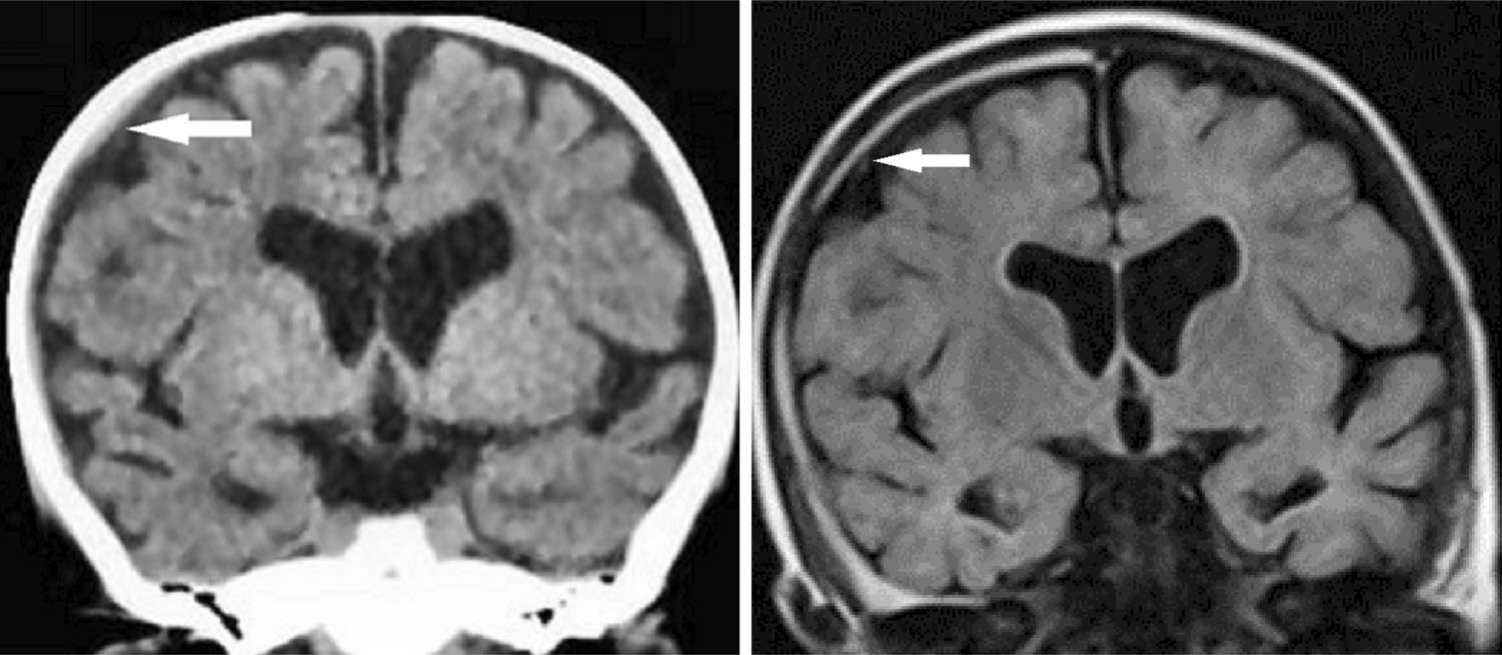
Case 3, male aged 7 months. Left: CT on arrival demonstrating tiny, high- and iso-dense SDH on the right cerebral convexity (arrow). Note the bilateral enlargement of the lateral ventricles and subarachnoid spaces. Right: MRI (FLAIR) 19 days after symptom onset demonstrating thin, film-like, high-intensity SDH on the right side (arrow). No mass effect or parenchymal abnormality was observed on the other sequences (not shown)
